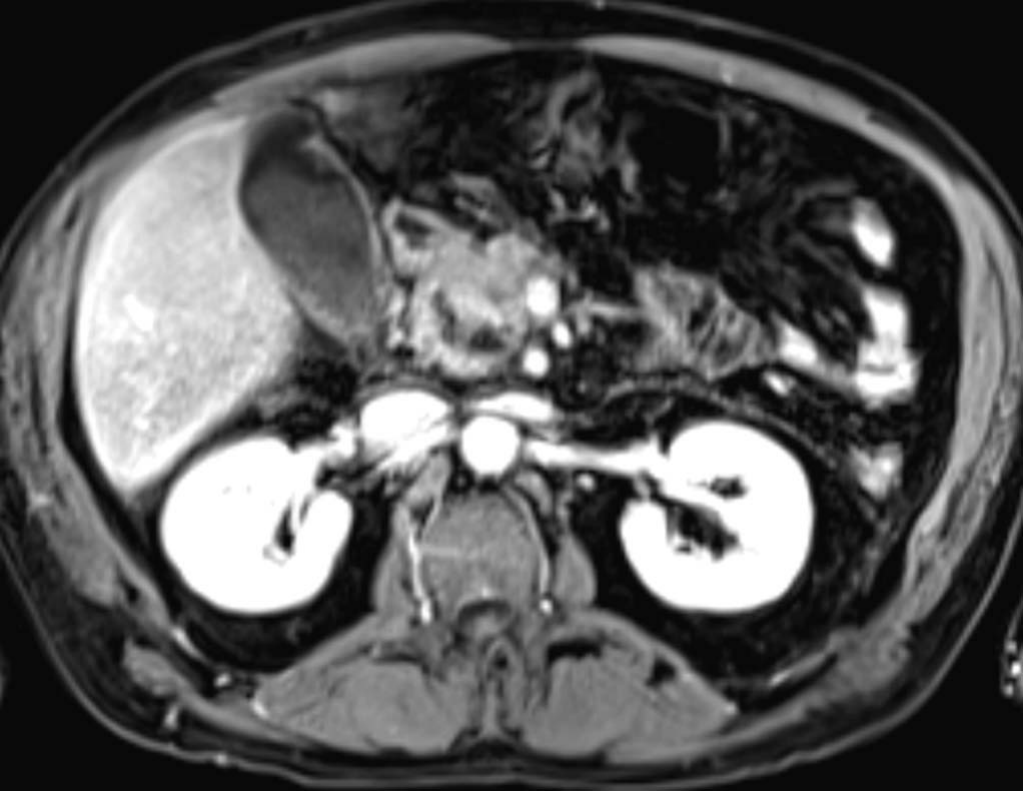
Magnetic resonance imaging of abdomen showing acute pancreatitis with necrotic collections containing fluid, debris, hemorrhage.
